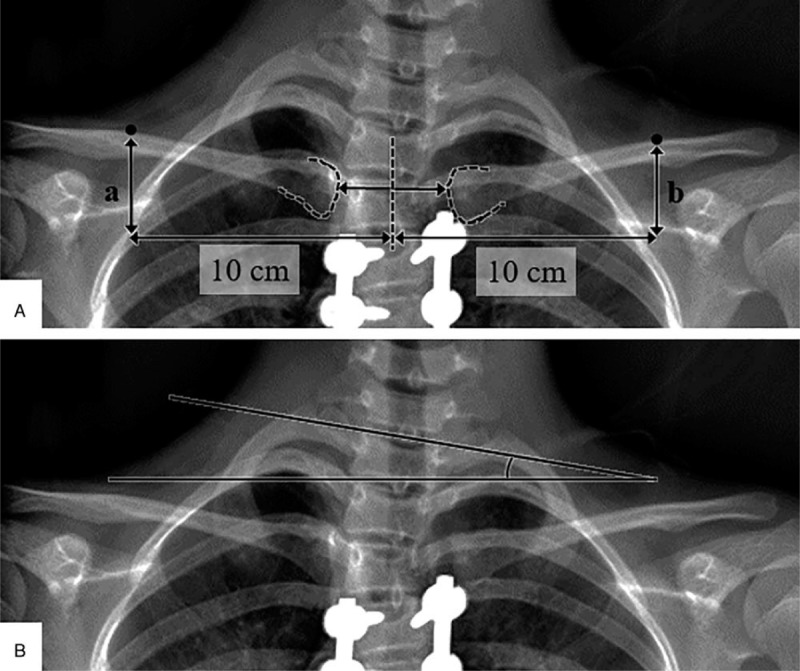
(A) Detailed measurement of radiographic shoulder height with calculation of the difference between points a and b (mm). (B) T1 tilt as the angulation between the T1 upper endplate and the horizontal line.
